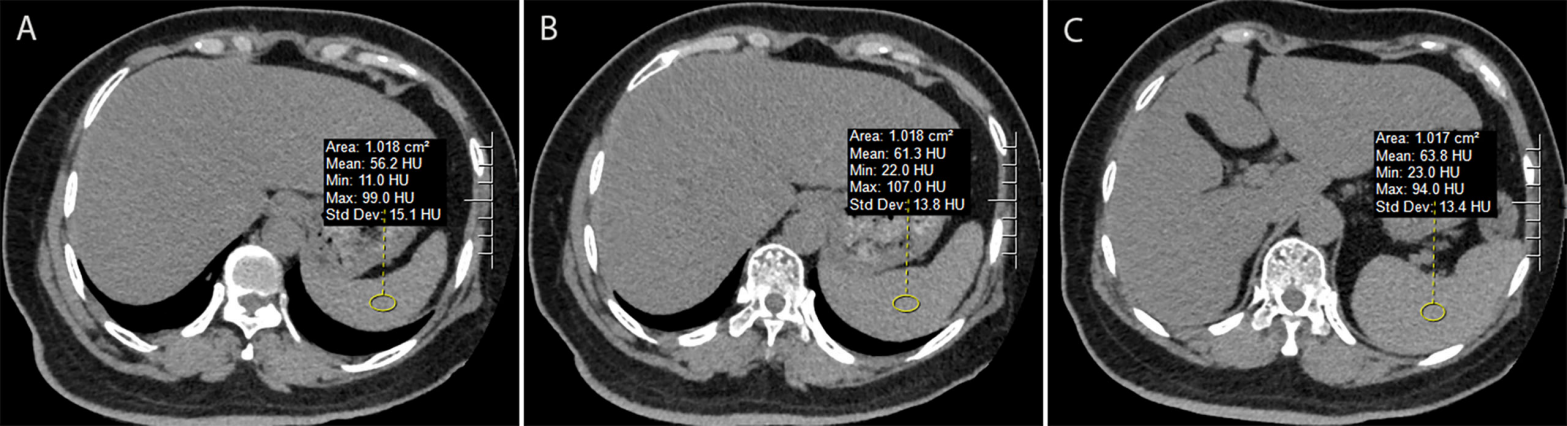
Measurement of splenic attenuation (SA) on unenhanced chest computed tomography using imaging Picture Archiving and Communication System. Three circular region-of-interests (about 1.0 cm^2^ in size) were placed in the upper (A), middle (B), and lower third (C) of the spleen respectively. The average of these three measurements was used for the SA value.
